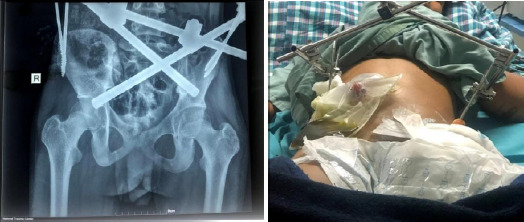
Postoperative status (A) X-ray anteroposterior view (B) External fixator with a colostomy bag.
